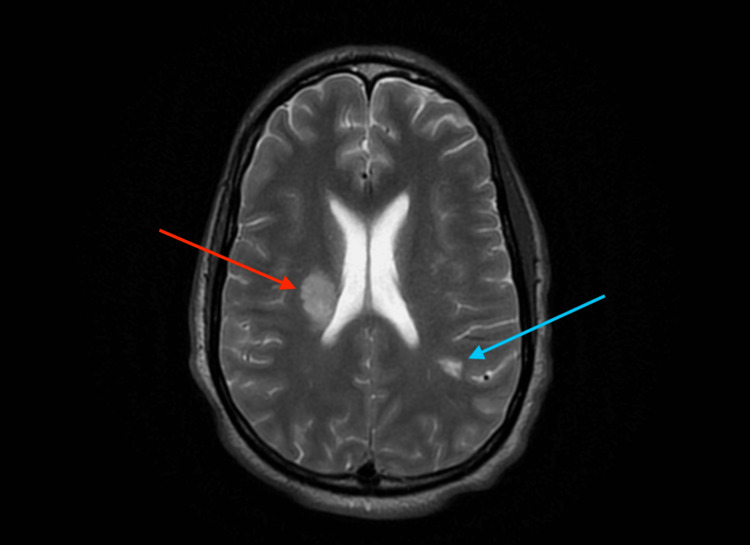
MRI brain demonstrating right periventricular, middle cerebral artery territory acute ischemic stroke (red arrow) with a left enhancing parietal lobe lesion (blue arrow).
